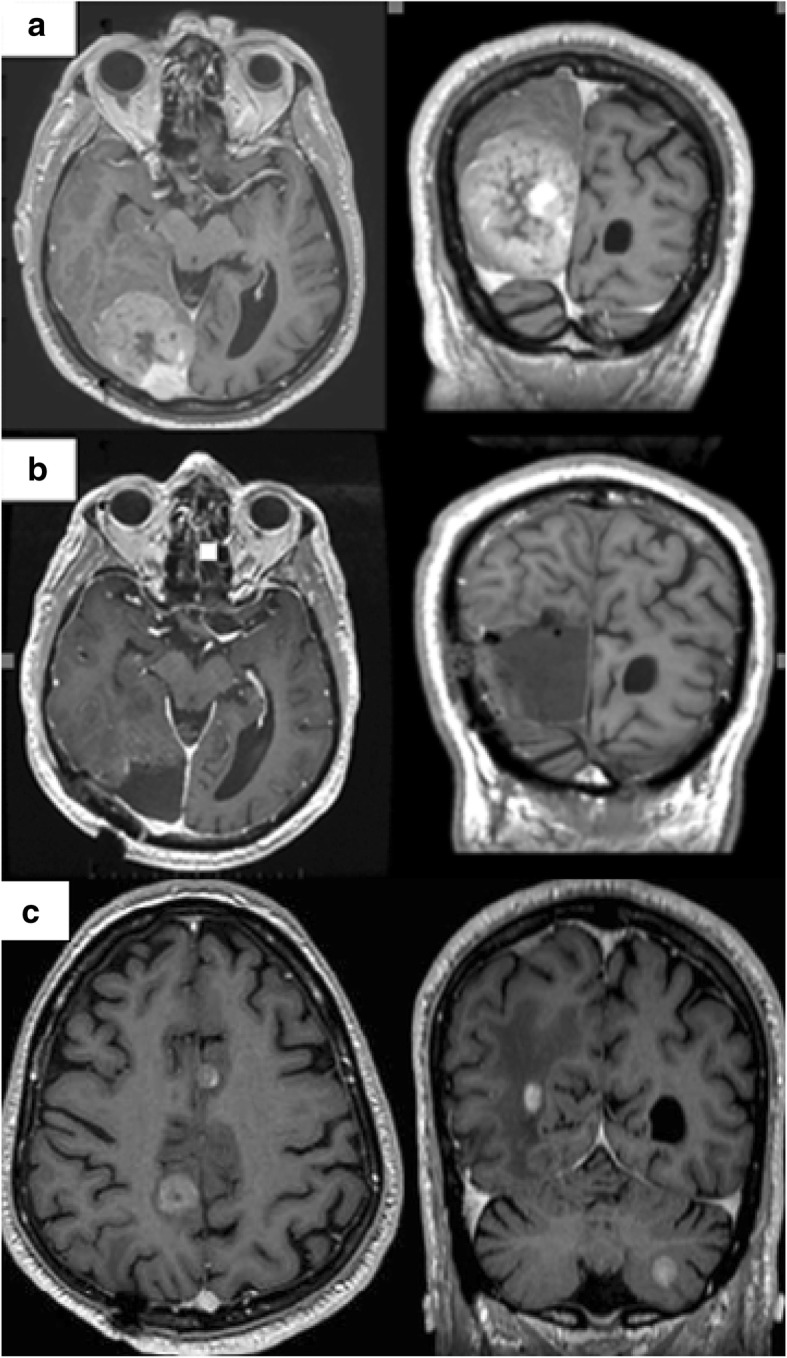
MRI-enhanced axial and coronal T1-weighted imagings. a An extra-axial lesion in the right occipital lobe with a mass effect of the posterior horn of the right ventricle. b Gross total resection of the right occipital meningioma. c Distant brain metastases of malignant meningioma
